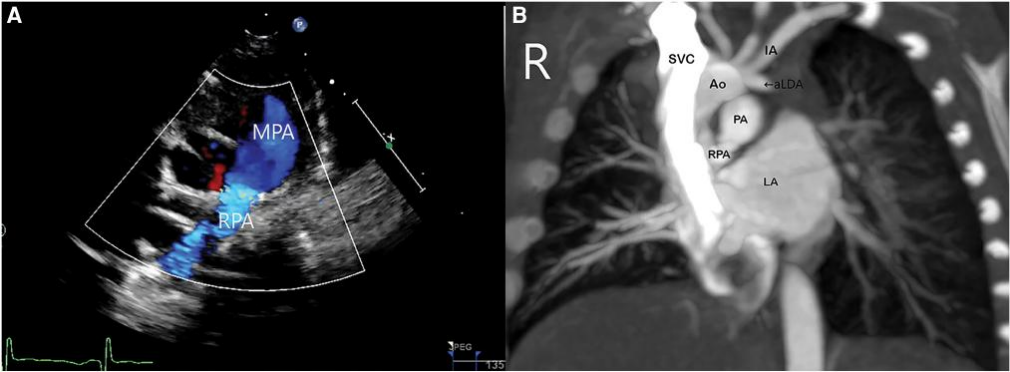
(*A*) Post-natal transthoracic echocardiography parasternal short axis view demonstrating flow from MPA to RPA. LPA was not visualized. (*B*) CT angiography demonstrating RPA arising from MPA. LPA again not visualized. A stump appearing from left IA can be seen, likely ampulla of left ductus arteriosus. MPA, main pulmonary artery; RPA, right pulmonary artery; SVC, superior vena cava; IA, innominate artery; Ao, aorta; aLDA, ampulla of left ductus arteriosus; PA, pulmonary artery; LA, left atrium.
